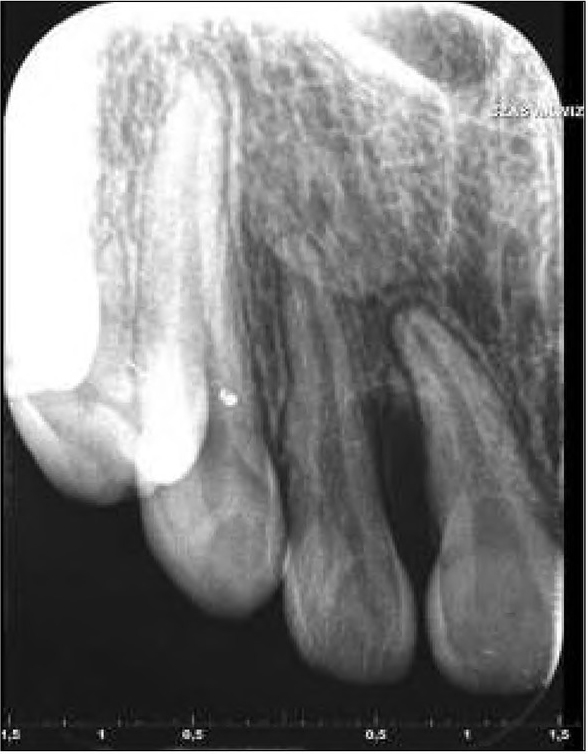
Intraoral x-ray. Bone loss between teeth 11-12. Rye. 1. Zdjęcie zębowe wewnątrzustne. Widoczna utrata kości pomiędzy zębami 11-12.
